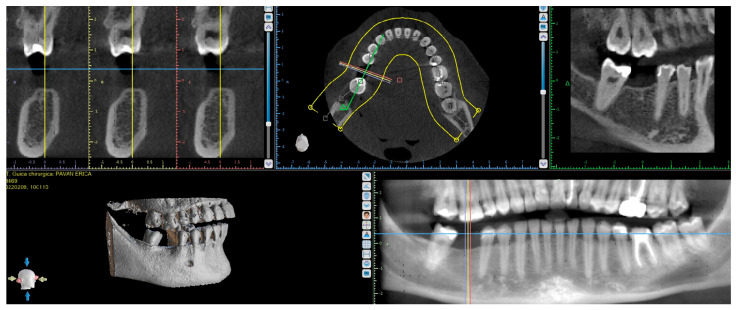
CBCT of patient before implant placement.
